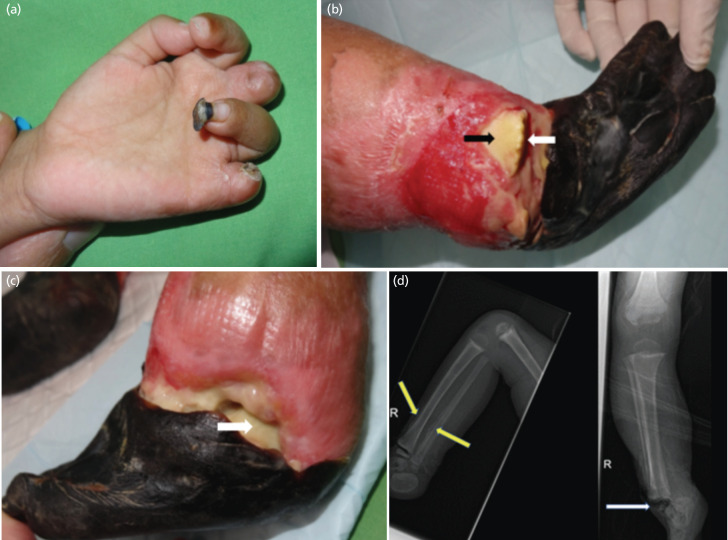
(a) Auto-amputation of the middle and little fingers. Dry gangrene at the tip of the ring finger. (b) Gangrene of the right foot. Necrosis of the physis resulted in the gap (white arrow) distal to the metaphysis (black arrow) of the right tibia. (c) Gangrene of the left foot. Soft tissue necrosis has exposed the ankle joint. The talus is visible (arrow). (d) Plain radiographs showing periosteal elevation of both tibia and fibula (yellow arrow). There is separation of the epiphysis from the metaphysis of the right tibia (white arrow).
